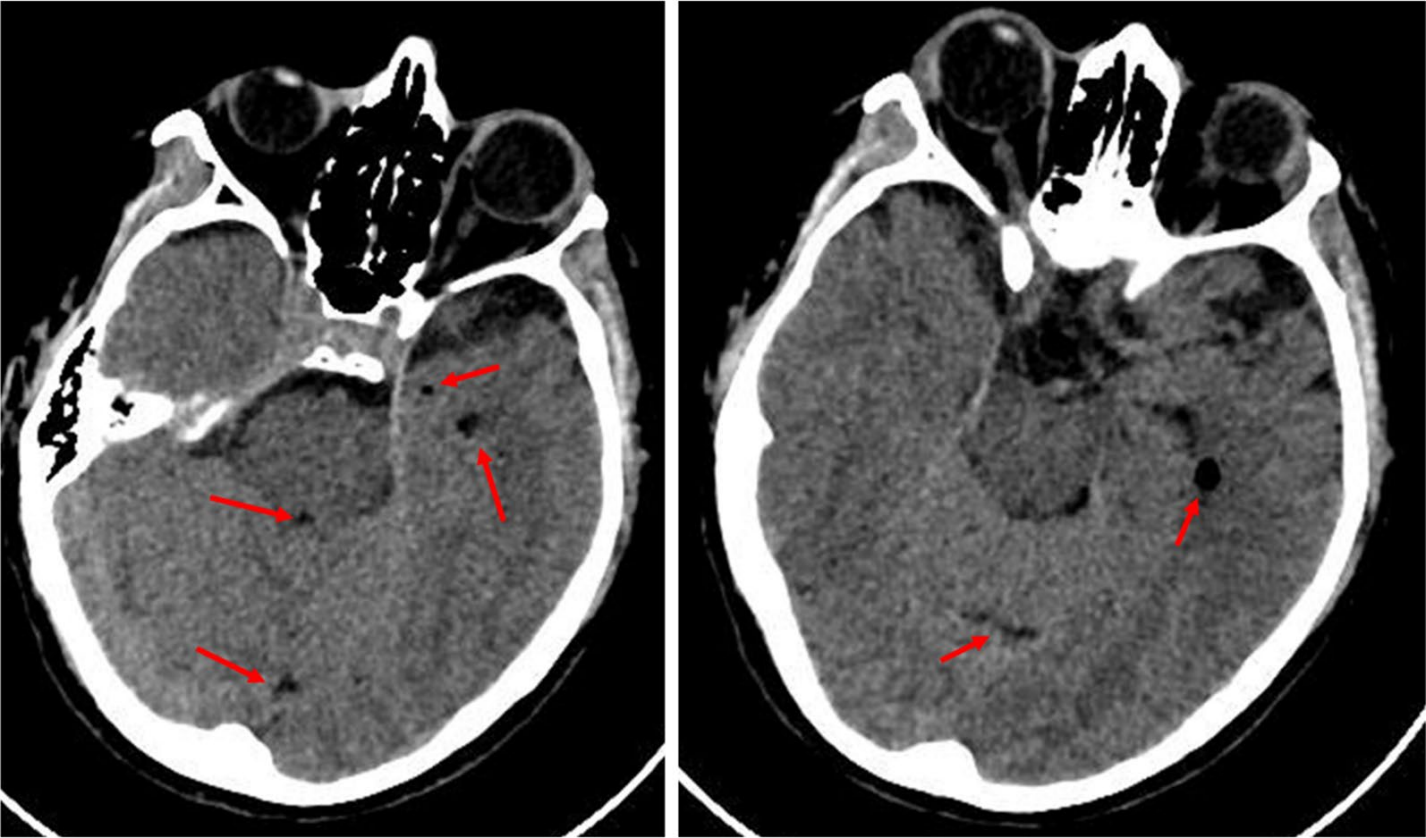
Basal CT scan indicated a thin frontal sub-thecal isodense layer associated with multiple small air bubbles (red arrows)
